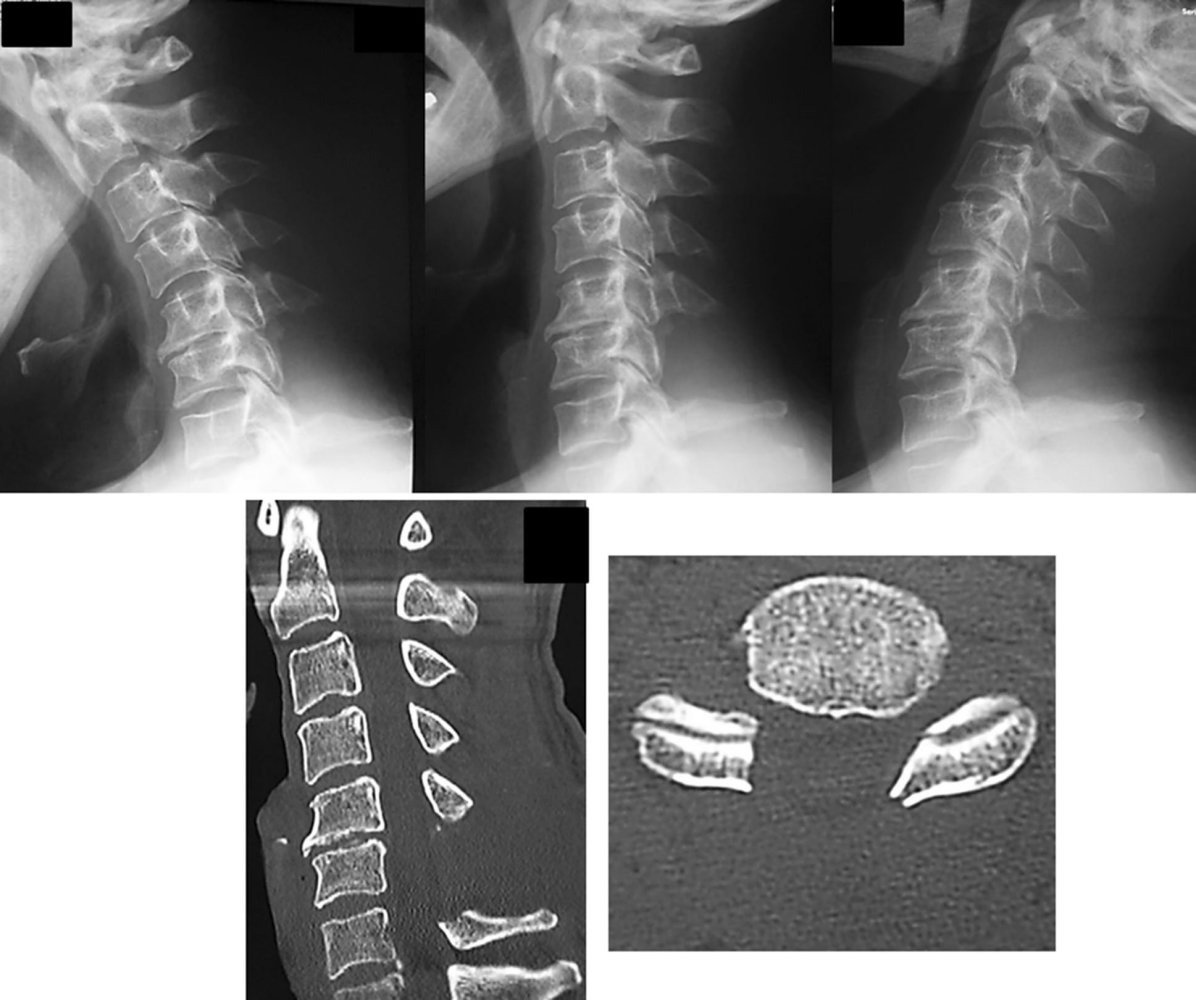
Postoperative plain radiograph and CT of the cervical spine. Post-operative radiograph of the cervical spine did not show each segmental instability. Moreover, post-operative CT scan showed complete resection of the tumor, and demonstrated that over 2/3 part of the facet was preserved
